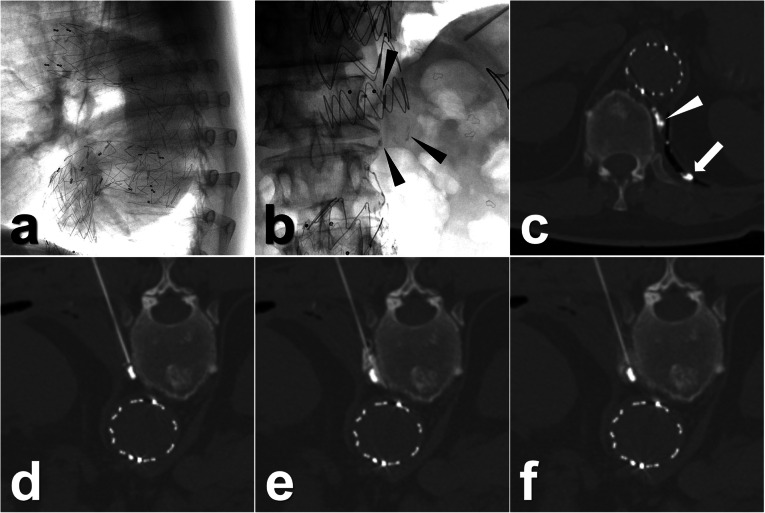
Images from a patient who underwent endovascular aneurysm repair procedures for the progressive thoracic aortic aneurysm 4 years before. a In the recent follow-up, the dislocation of the thoracic grafts was found. Then, aortic replacement surgery was performed. After the surgery, the left chylothorax was diagnosed with a daily drainage output of around 1,500 mL/day. b In the TL, a small amount of iodinated oil extravasation was observed (black arrowheads) near the left crural diaphragm. c Also, CT definitely showed the extravasation from the ruptured thoracic duct (white arrowhead) into the pleural cavity (white arrow). Eight days later, the percutaneous ALVS was performed owing to there being no obvious regression of the drainage. d During the procedure, the needle puncture was close to the afferent thoracic duct. e Then, 1 mL of contrast fluid was injected that showed an ideal distribution around the thoracic duct. f Afterwards, 2.0 mL 95% ethanol was injected; the final CT scan showed a good distribution of the prior injected contrast fluid surrounding the target lymphatic vessel. From then on, the chylothorax output gradually reduced and completely ceased after 1 week. Then, the drainage was removed and the patient was discharged on the next day. *ALVS* Afferent lymphatic vessel sclerotherapy, *CT* Computed tomography, *TL* Transpedal lymphangiography
